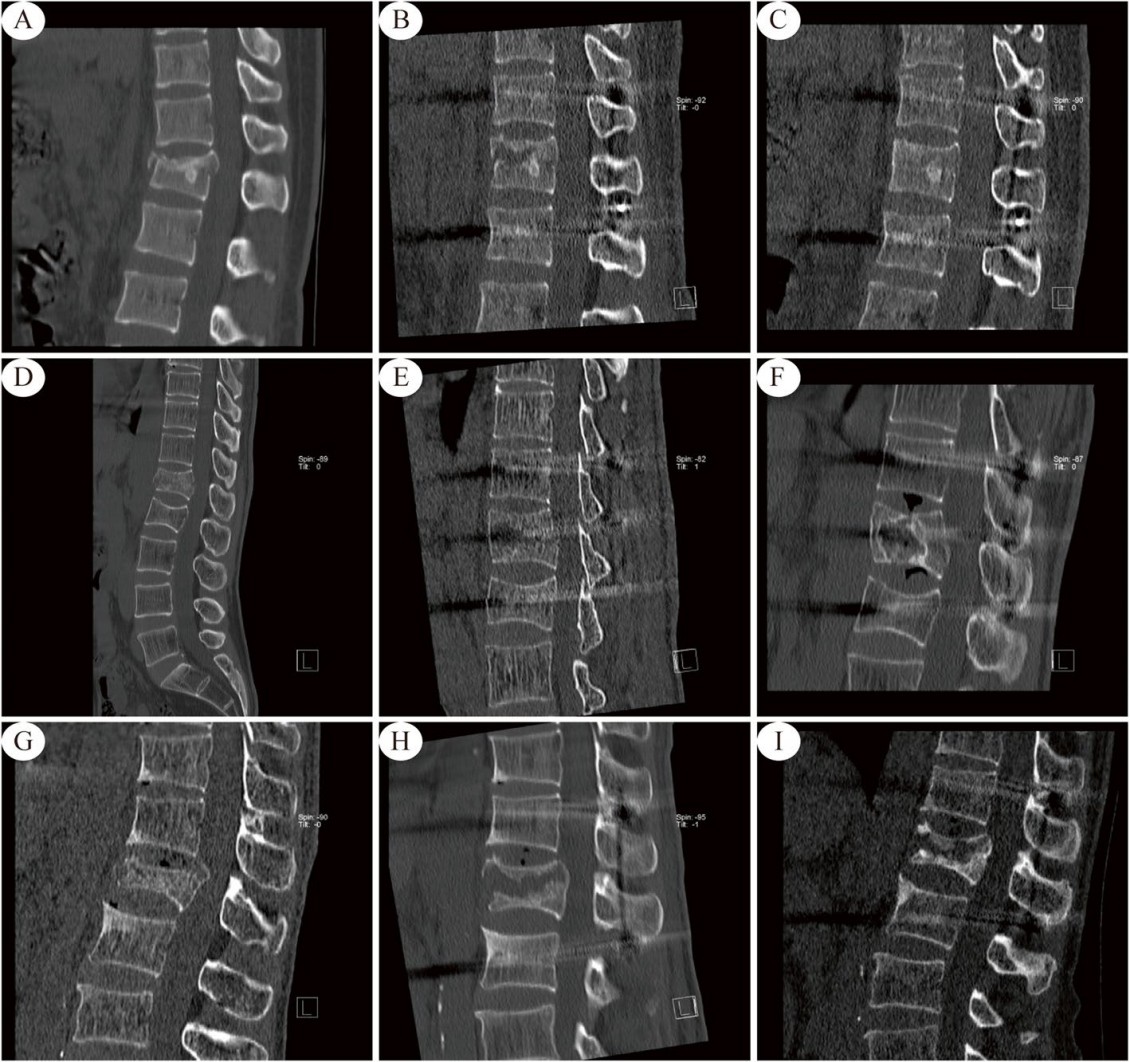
The preoperative, 3 days postoperative and 1-year postoperative CT sagittal views are shown from left to right. A, B, C Shrinkage or healing type. Female ,36 years. L1 Compression fracture. Traffic Accidents. D, E, F Enlarged or end plate collapse type. Male,49 years. L1 Burst fracture. High fall injuries. G, H, I Shrinkage with endplate collapse type. Male ,62 years. L1 Burst fracture. High fall injurie
